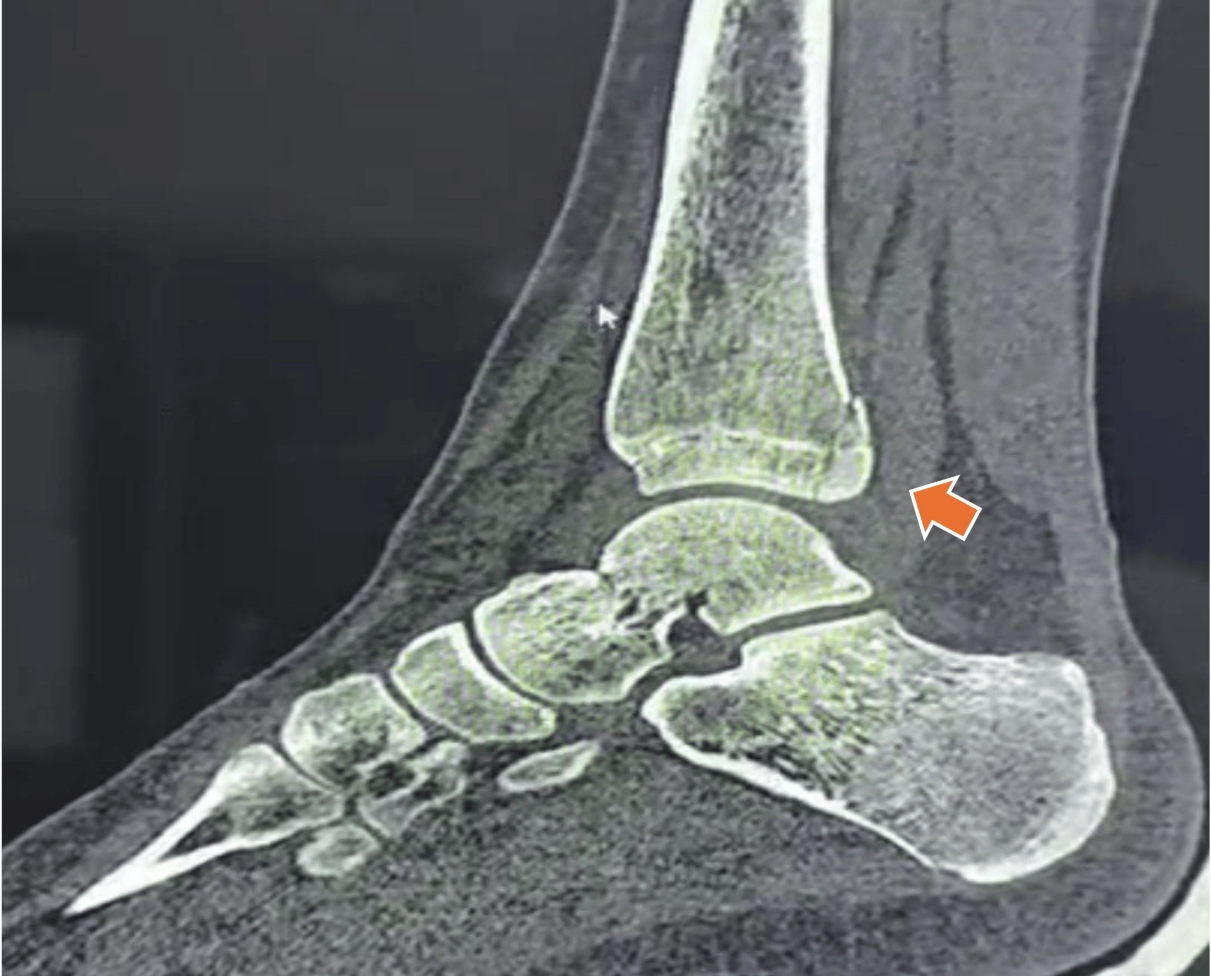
Posterior malleolus fracture, sagittal CT. The orange arrow indicates the posterior malleolus fracture line. The Tillaux fragment is located anteriorly, as demonstrated on the axial CT.
